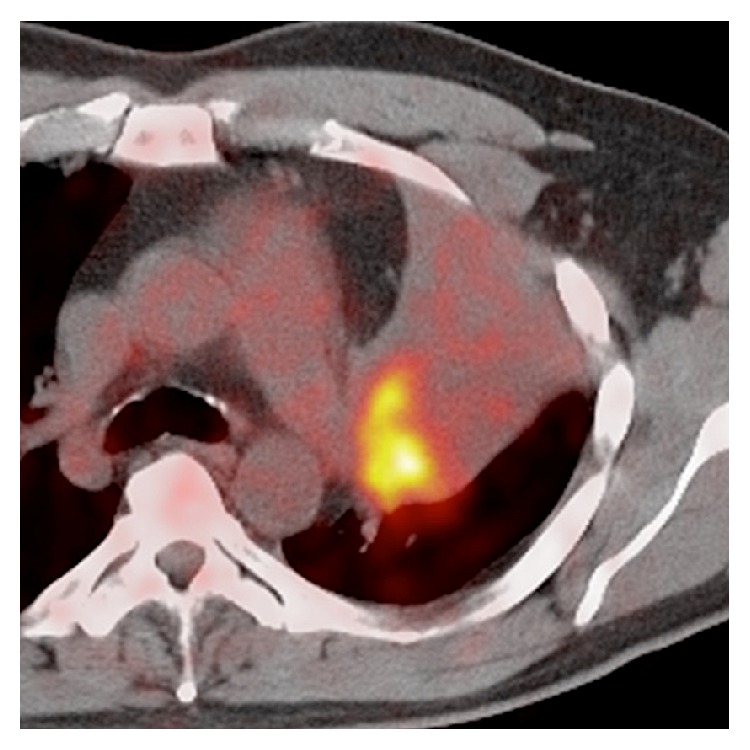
Axial PET/CT image demonstrates avid FDG uptake by the left upper lobe mass.
